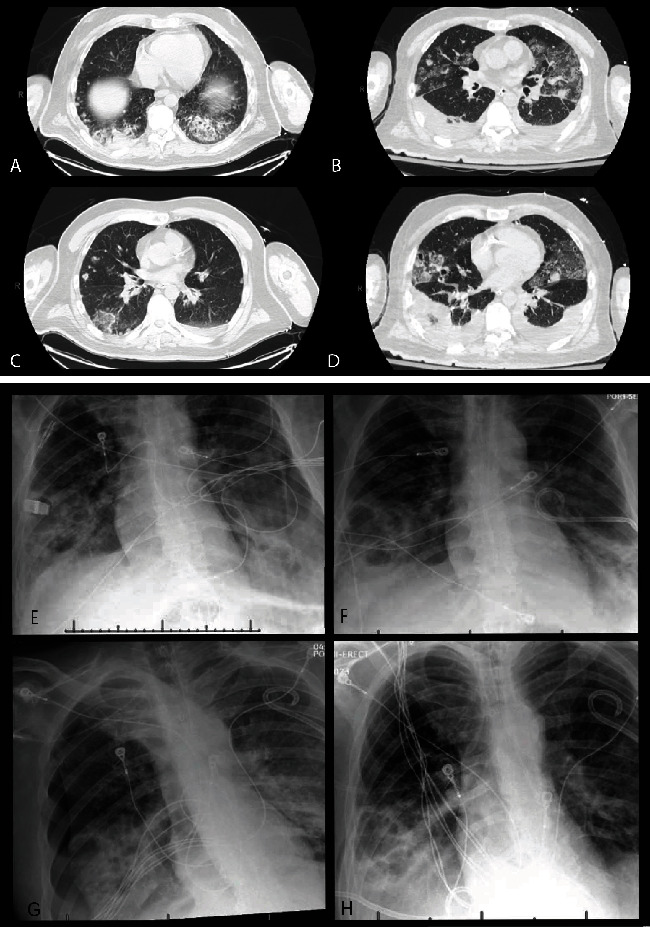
(A, B) Initial CT chest images showing bilateral nodular lesions consistent with embolic phenomenon along with basilar ground glass opacities. (C, D) Repeat CT chest images showing progression of nodular embolic lesions into cavitary lesions. (E, F) Right pneumothorax—pre- and postchest tube placement. (G, H) Left pneumothorax—pre- and postchest tube placement.
